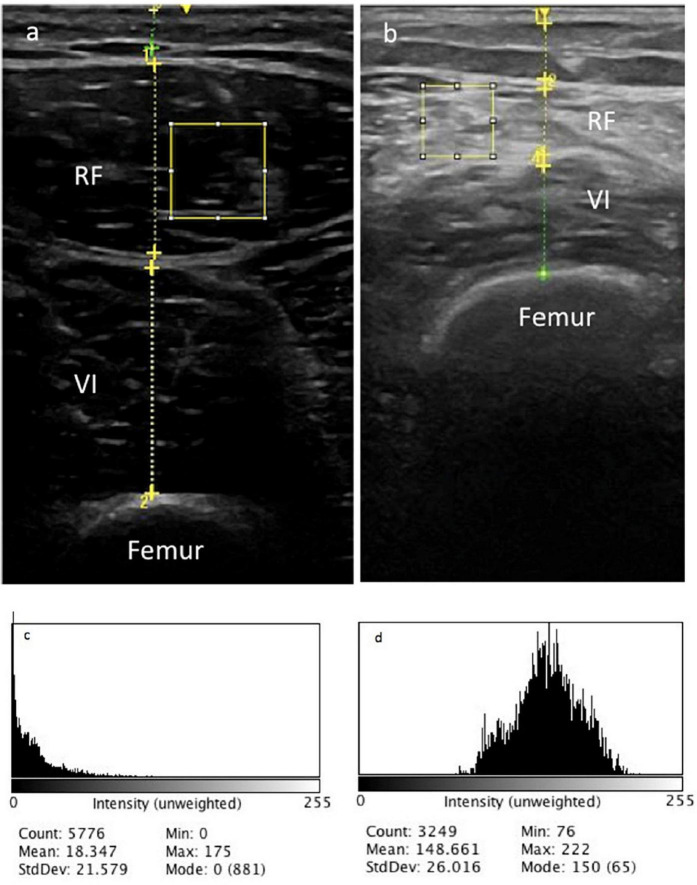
Muscle thickness of right *rectus femoris* (RF) and *vastus intermedius* (VI) muscles ultrasonography of two different long COVID patients (a,b). The selected area represents the region of interest (ROI). Histogram of RF echo intensity of patient a (c) and patient b (d).
